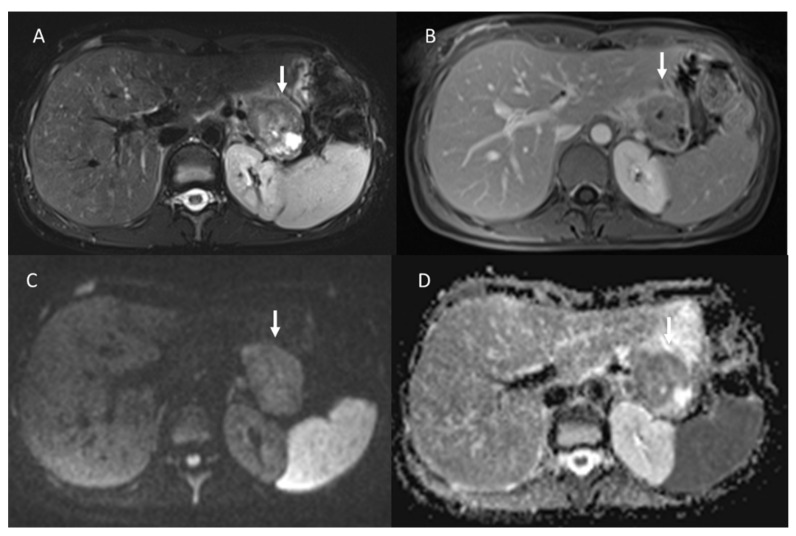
MRI assessment of pancreatic NEN. In T2-W (A) sequence, the lesion (arrow) is inhomogeneous and hypo–hyperintense due to fibrotic component. This feature is evident in late phase (B) of contrast study (arrow). In DWI ((C): b800 s/mm^2^), the lesion (arrow) shows restricted diffusion with hypointense signal in ADC map (arrow in (D)).
